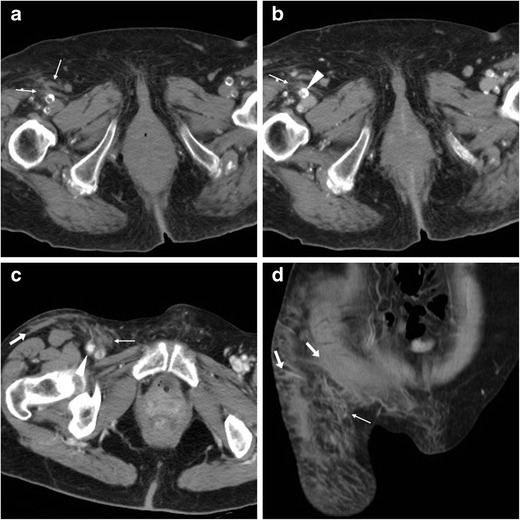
Expected CT appearance following uncomplicated percutaneous access to the right common femoral artery (CFA) in two different patients. In an 82-year-old diabetic female patient, 48 h after coronary angiography (CA) and coronary artery stenting, focused precontrast (A) and arterial-phase (B) CT images showed mild fat stranding and fluid (thin arrows) along the probable puncture tract, abutting the ventral aspect of the calcified but patent CFA (arrowheads). The day after CA, in a 90-year-old female patient, CT-angiography (C, D) showed similar changes (thin arrows) surrounding the CFA (arrowhead) and high-attenuation fascial blood (arrows), which did not require treatment
